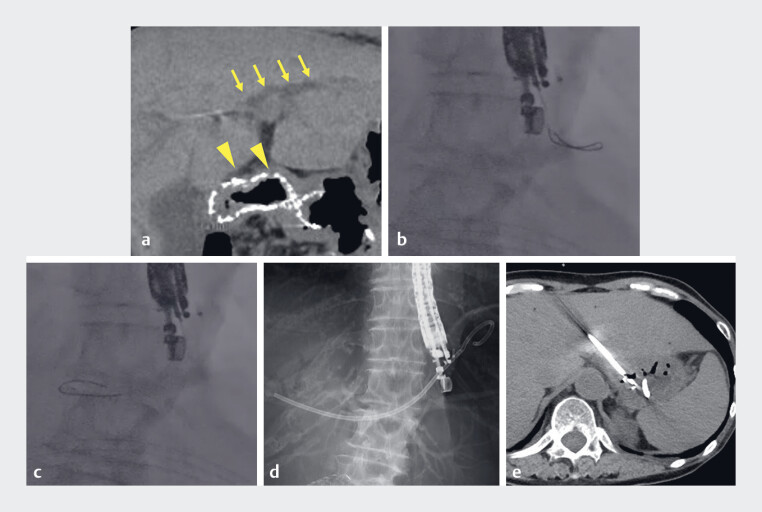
Computed tomography (CT) and cholangiography images for case 1.
